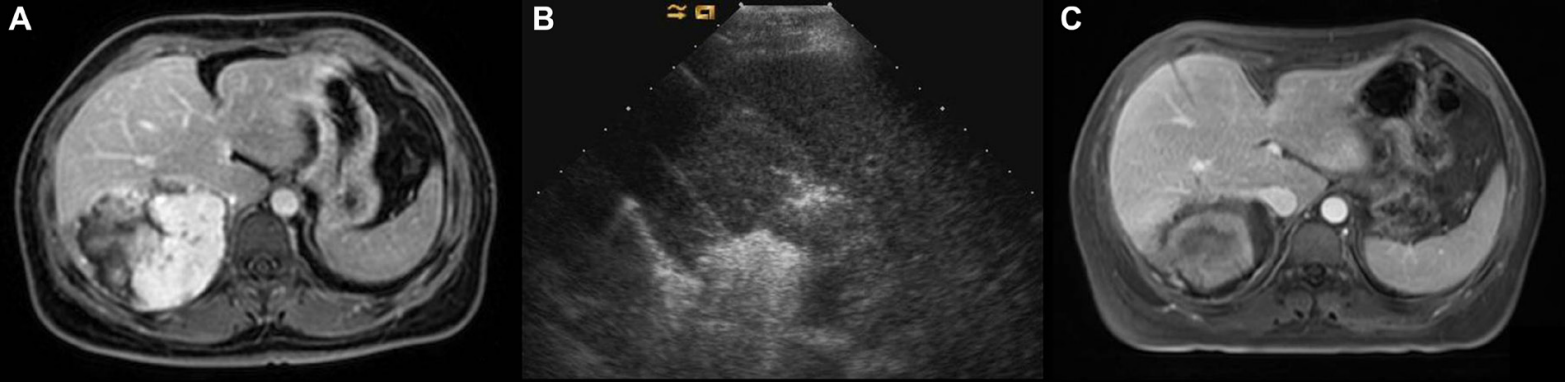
Images in a 39-year-old-woman who underwent MWA for hepatic cavernous hemangioma 10.4 cm in diameter (A) Preoperative contrast-enhanced MRI showed the hemangioma was hyperenhanced in portal vein phase in right lobe. (B) US image showed the two antennae were placed into the tumor and ablated simultaneously. (C) Postoperative contrast-enhanced MRI showed complete necrosis of the tumor and and the maximum diameter was reduced to 7.8 cm 3 days after MWA.
